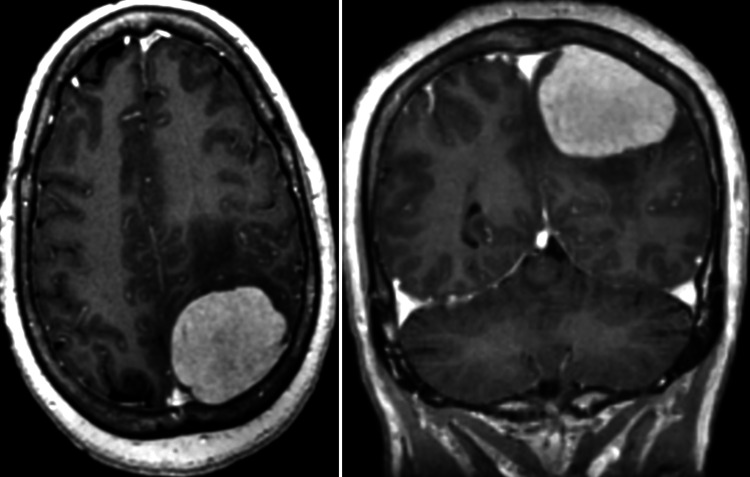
Magnetic resonance imaging (MRI) of the brain with contrast. MRI of the brain with contrast shows a homogeneously enhancing and well-defined dural-based mass at the left parietal convexity, causing mass effect upon the underlying brain parenchyma. The lesion measures 5 × 4 × 3.3 cm and is associated with mild hyperostosis of the overlying parietal bone. The mass demonstrated a low signal on the non-contrast T1-weighted images and intermediate signal intensity on T2-weighted images (not shown). There is also left atrial effacement and minimal vasogenic edema.
